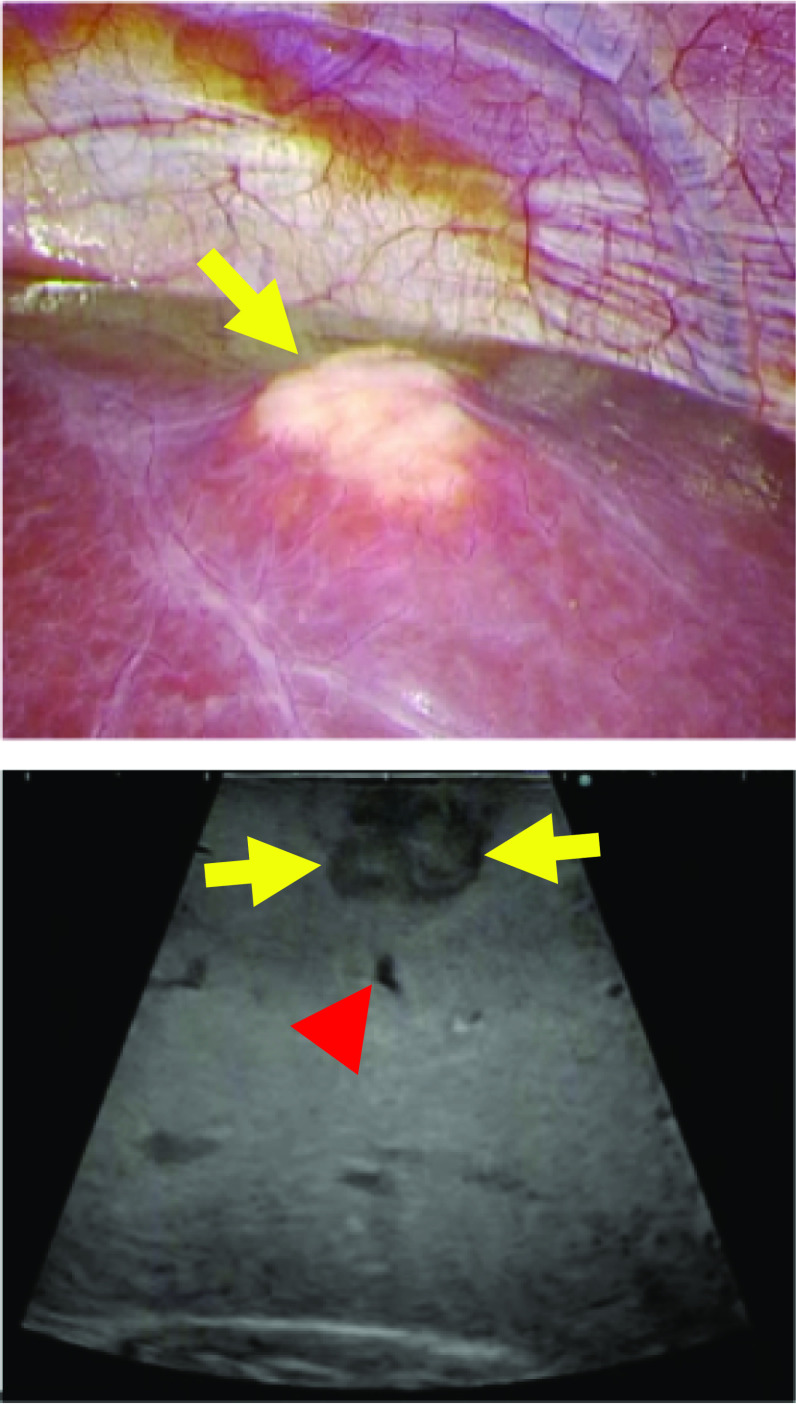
In the pre-operative work-up of patients with liver tumors. Laparoscopy prior to hepatectomy revealed that the tumor was located in segment 7 on the liver surface (Yellow arrow). Intraoperative ultrasonography confirmed metastasis in segment 7. It was 18 mm in size and distant from the main glissons, right hepatic vein, middle hepatic vein, and vena cava (Yellow arrow). There was no peripheral glissons at the base of the tumor and a peripheral hepatic vein 2 mm in diameter was identified (Red arrowhead)
